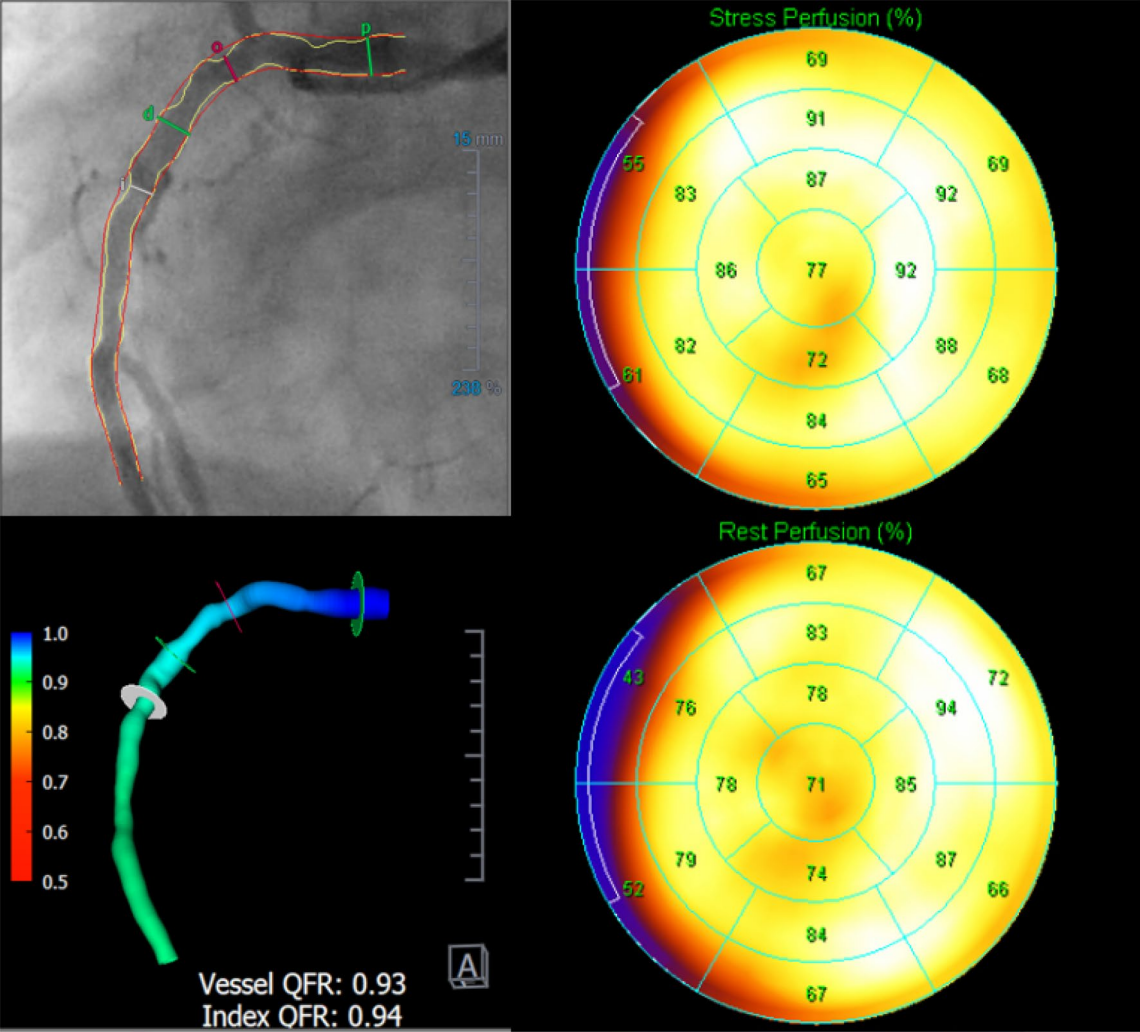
Study procedure. Example of study procedure showing a vessel visually estimated as > 90% stenosis with normal MPS assessment. Right side represents the MPS results, upper left is QCA, lower left show QFR results in a flow distribution map of the vessel. *MPS* myocardial perfusion scintigraphy, *QCA* quantitative coronary angiography, *QFR* quantitative flow ratio
